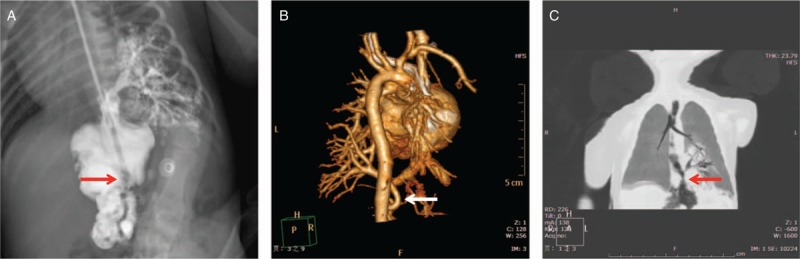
Picture from Case 1. A, Upper gastrointestinal tract radiography (arrow indicating an abnormal esophagobronchial passage). B, CT 3D reconstruction (arrow indicating an abnormal blood supply). C, CT esophagobronchial 3D reconstruction (arrow indicating an abnormal esophagobronchial passage). 3D = three-dimensional, CT = computed tomography.
